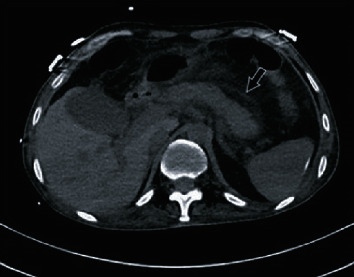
Abdominal noncontrast-enhanced computed tomography in a 44-year-old man recovering from severe coronavirus disease 2019 pneumonia with signs of acute pancreatitis, including inflammatory changes in peripancreatic fat in the pancreas (white-framed arrow).
